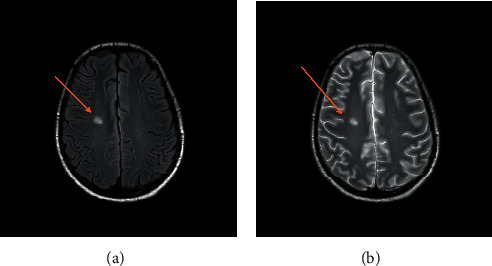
Flair MR image showing high signal intensity (left image orange arrow) and corresponding T2-weighted image showing an area of high signal intensity (right image orange arrow).
